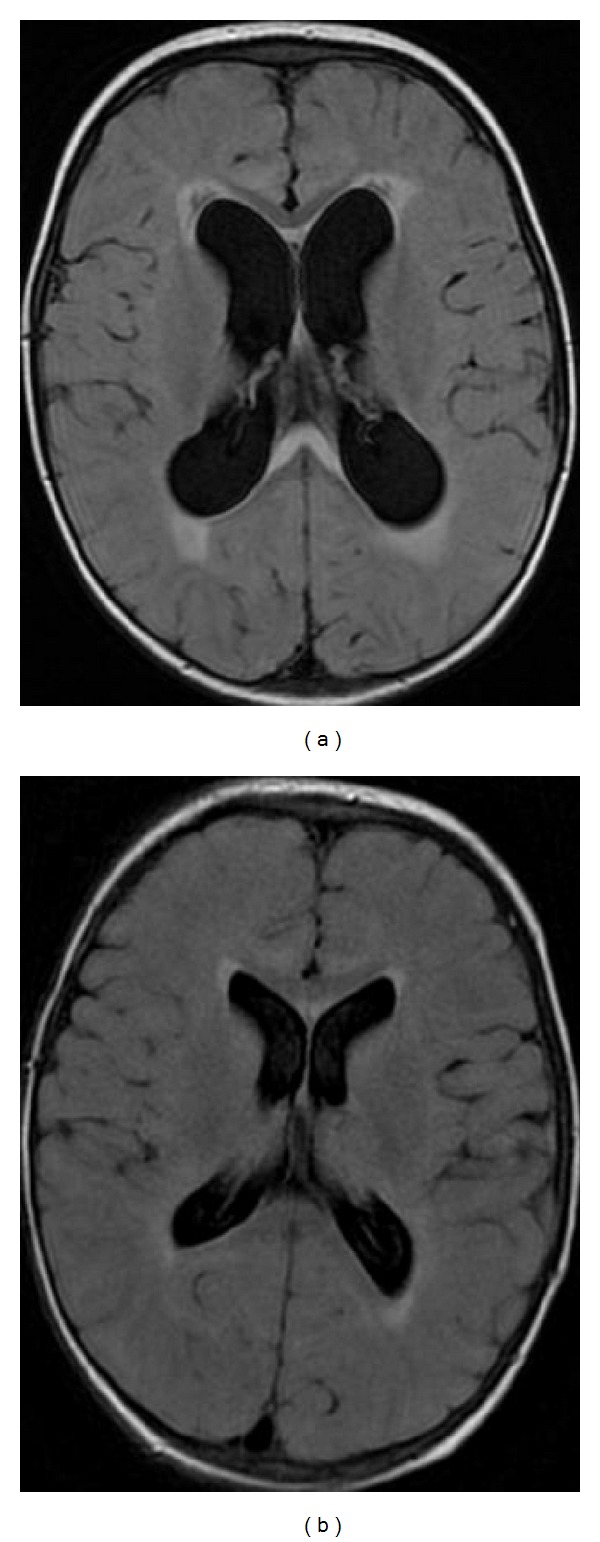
FLAIR, axial plane. (a) Five year-old boy with active hydrocephalus. (b) Three months later the ventricles are narrower and normalization of callosal signal intensity is observed.
